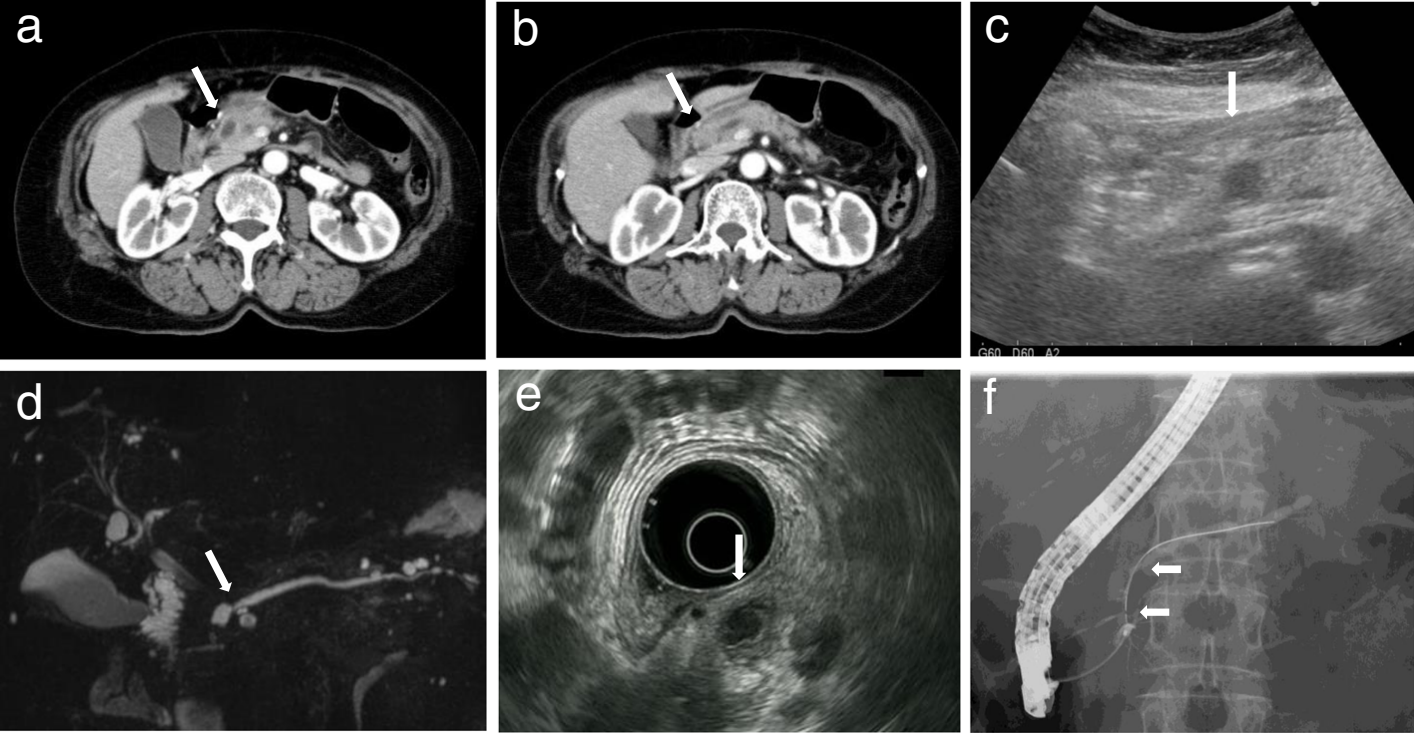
a, b CT showed a cystic mass in the pancreatic head (arrow). Slight fat stranding suggested that inflammation was localized to the pancreatic head. The MPD was dilated from the body to the tail (arrow). c The cystic mass was demonstrated as a hypo-echoic area under US (arrow). d MRCP showed that the MPD was obstructed by the cystic mass (arrow). e EUS revealed that the masswas 15 mm and comprise of both solid and cystic components (arrow). f ERP showed the MPD was obstructed for a 12-mm length (arrow). There was no obvious communication between the cystic mass and the MPD
